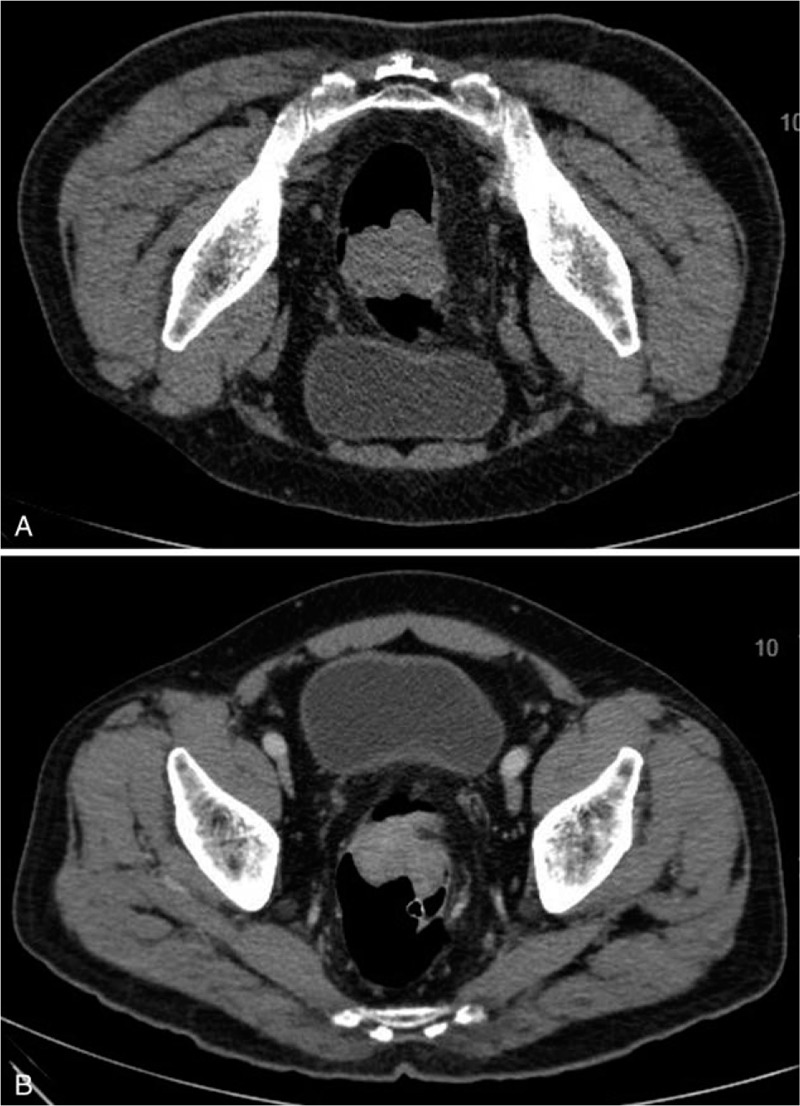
(A and B) CT colonography showing rectal adenocarcinoma.
